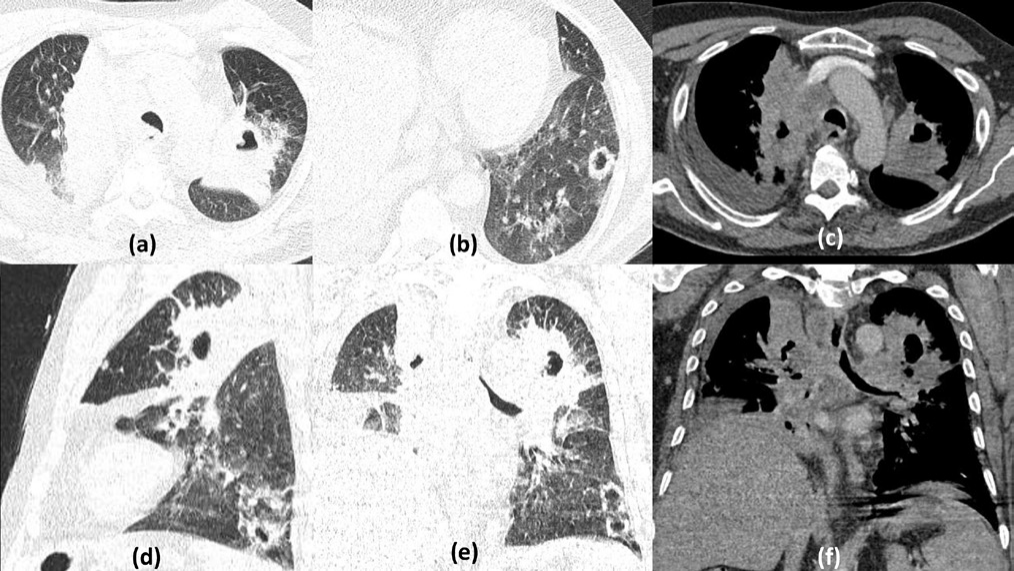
Primary lung cancer with multiple cavitating nodules and COVID19 infection: 60-year-old smoker with hemoptysis, shortness of breath and fever tested positive for COVID19 virus. Chest CT sections show a large mass lesion in the left parahilar region and upper lobe showing areas of central cavitation having irregular inner walls with spiculated outer margins, with extension into the fissure (sagittal section d). Multiple cavitating nodules are seen the lower segments. Focal areas of ground glass opacities are seen in the left lower lobe consistent with COVID19 (zoomed axial image b). On the contrast enhanced sections (axial c and coronal f) the mass demonstrates heterogenous enhancement. Also seen are multiple bulky necrotic mediastinal lymph nodes causing collapse of contralateral upper lobe and right-sided pleural effusion.
